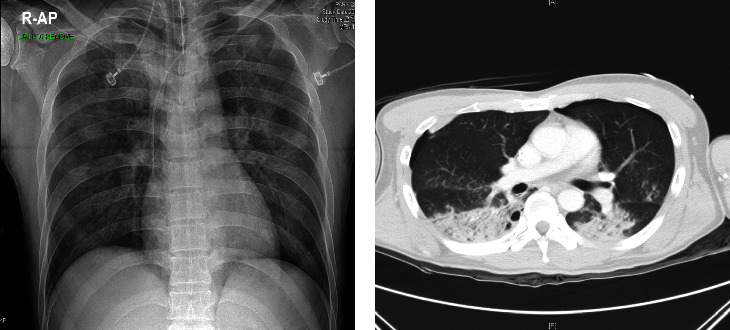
Imaging findings of a 48-year-old male traffic accident patient. (a) Lung contusion can be seen on the chest anteroposterior (AP) radiograph. (b) Lung contusion and pneumothorax can be seen on the chest computed tomography image. Pneumothorax was not visible in the chest AP radiograph.
